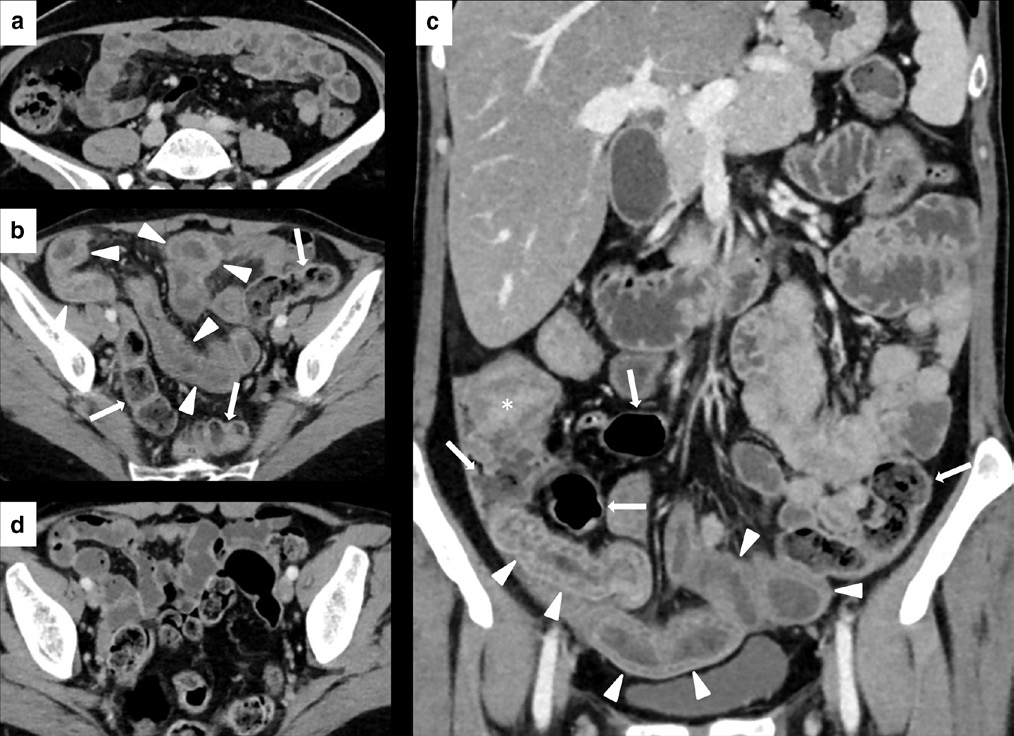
Imaging of ileitis without colitis in a 51-year-old female with metastatic breast cancer treated with Atezolizumab. Contrast-enhanced CT (CECT) at baseline did not show abnormalities of the last ileal loops wall (A). After 3 month of therapy, CECT showed a marked wall thickening, edema, and stratification involving the distal ileal loops (white arrowheads in B and C), without signs of colitis (white arrows in B and C, which show a regular colonic wall). After treatment discontinuation, CECT showed a remission of the ileitis (D). White asterisk in C shows the ileocecal valve.
